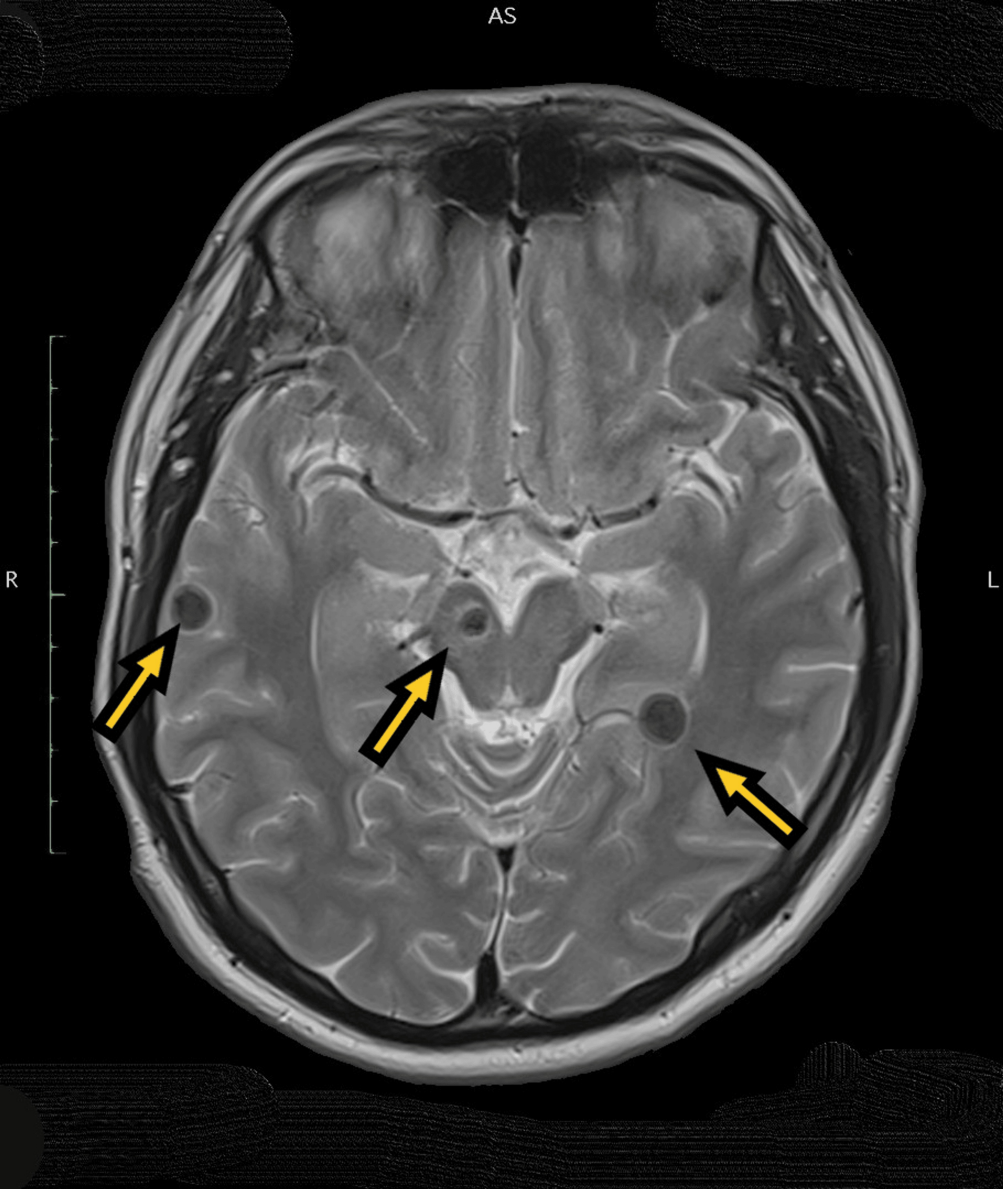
MRI of the brain, T2-weighted axial section, showing bilateral temporal and right midbrain cysts with hypointense cystic wall and minimal perilesional edema (yellow arrow).
